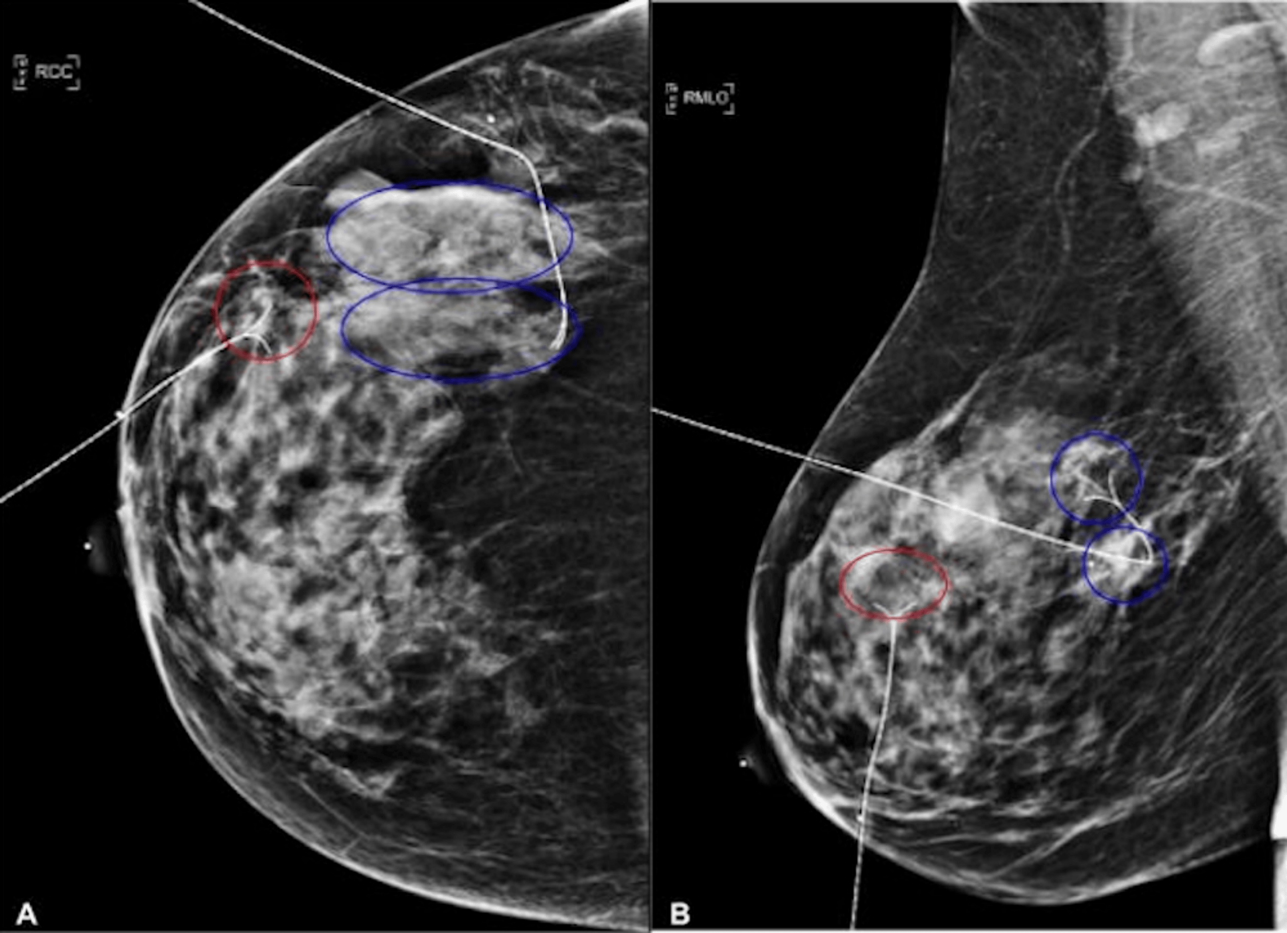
Control mammography post ultrasound-guided wire insertion (A) A right craniocaudal digital mammogram demonstrates two radiopaque localization hook-wires (Bard™ DuaLok™, 20 G × 7.7 cm; Becton, Dickinson and Company, Franklin Lakes, New Jersey, United States) within the breast parenchyma. One wire is directed toward the medial/anterior third of the breast, marking a solitary malignant nodule (encircled in red) in the anterior interquadrant; the second wire is oriented toward the lateral/posterior third, localizing two adjacent malignant nodules (encircled in blue) in the posterior interquadrant. (B) A right mediolateral oblique digital mammogram of the right breast demonstrates two radiopaque localization hook-wires (Bard™ DuaLok™, 20 G × 7.7 cm) traversing the breast parenchyma. One wire is directed toward the anterior third of the breast, marking a solitary malignant nodule (encircled in red) in the anterior interquadrant; the second wire courses into the posterior third, localizing two adjacent malignant nodules (encircled in blue) in the posterior interquadrant. The pectoralis muscle is seen posteriorly.
